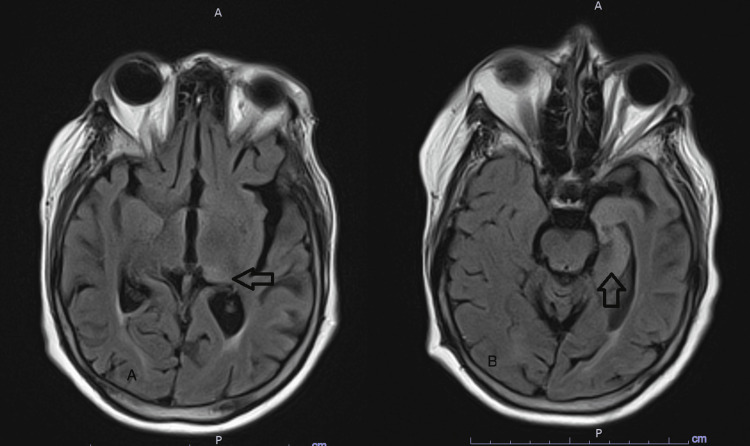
Initial MRI brain findings MRI brain showing areas of hyper-intensity on fluid attenuation inversion recovery (FLAIR) sequence in left thalamus (arrow in A) and left hippocampus (arrow in B).
